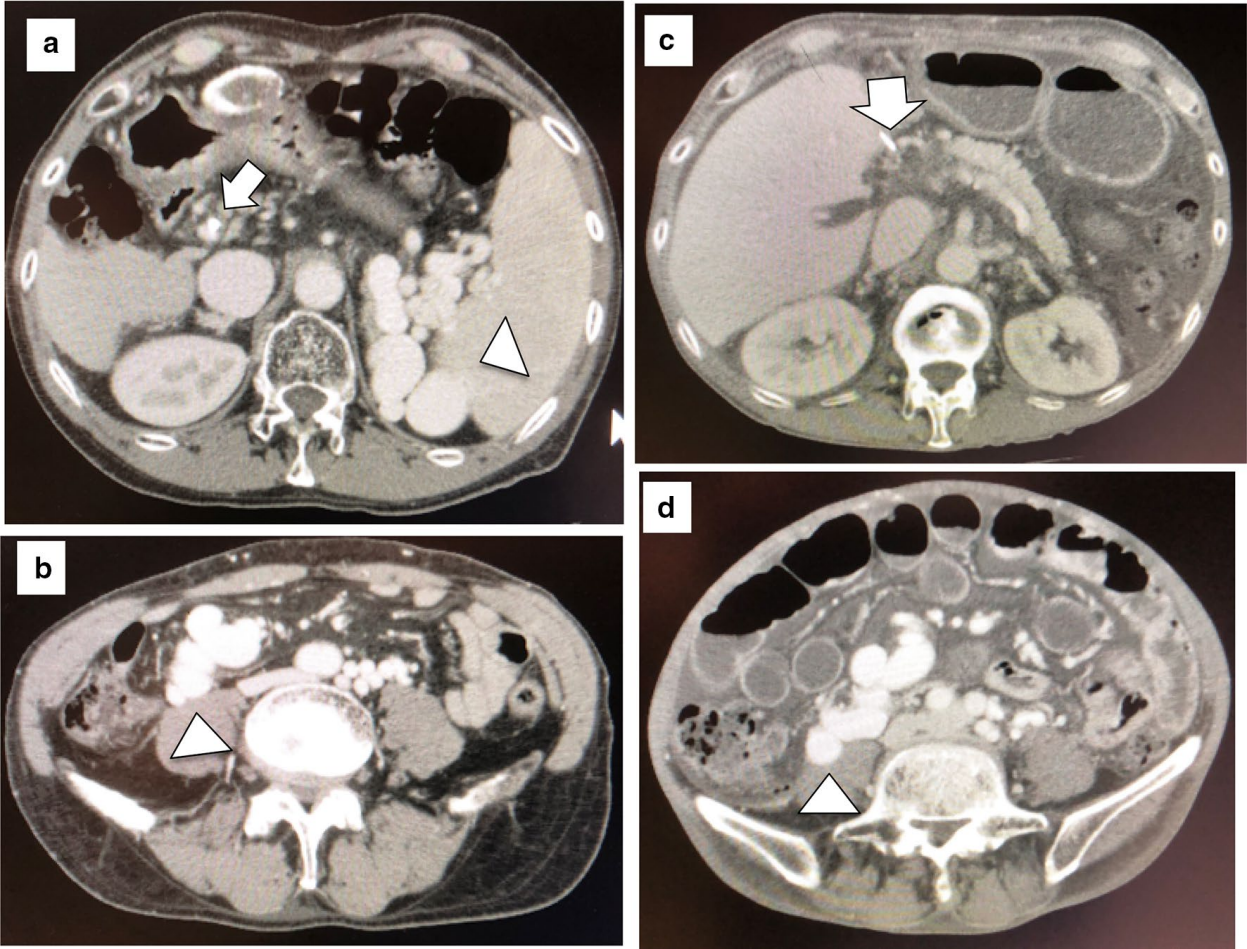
Representative case of a patient with a huge portosystemic shunt. a, b Pre-LDLT: enhanced CT shows huge splenorenal (arrow head) and mesocaval (arrowhead) shunts. The portal vein was atrophic (arrow). c, d 3 months after LDLT with splenectomy. Enhanced CT reveals a patent mesocaval shunt (arrowhead). The portal vein (arrow) was thrombosed. The splenorenal shunt was closed with simultaneous splenectomy
